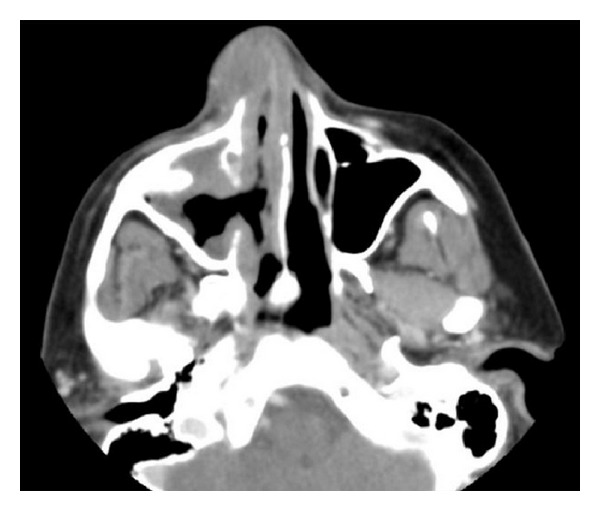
Axial contrast enhanced CT scan redemonstrating subcutaneous nasal lesion.
